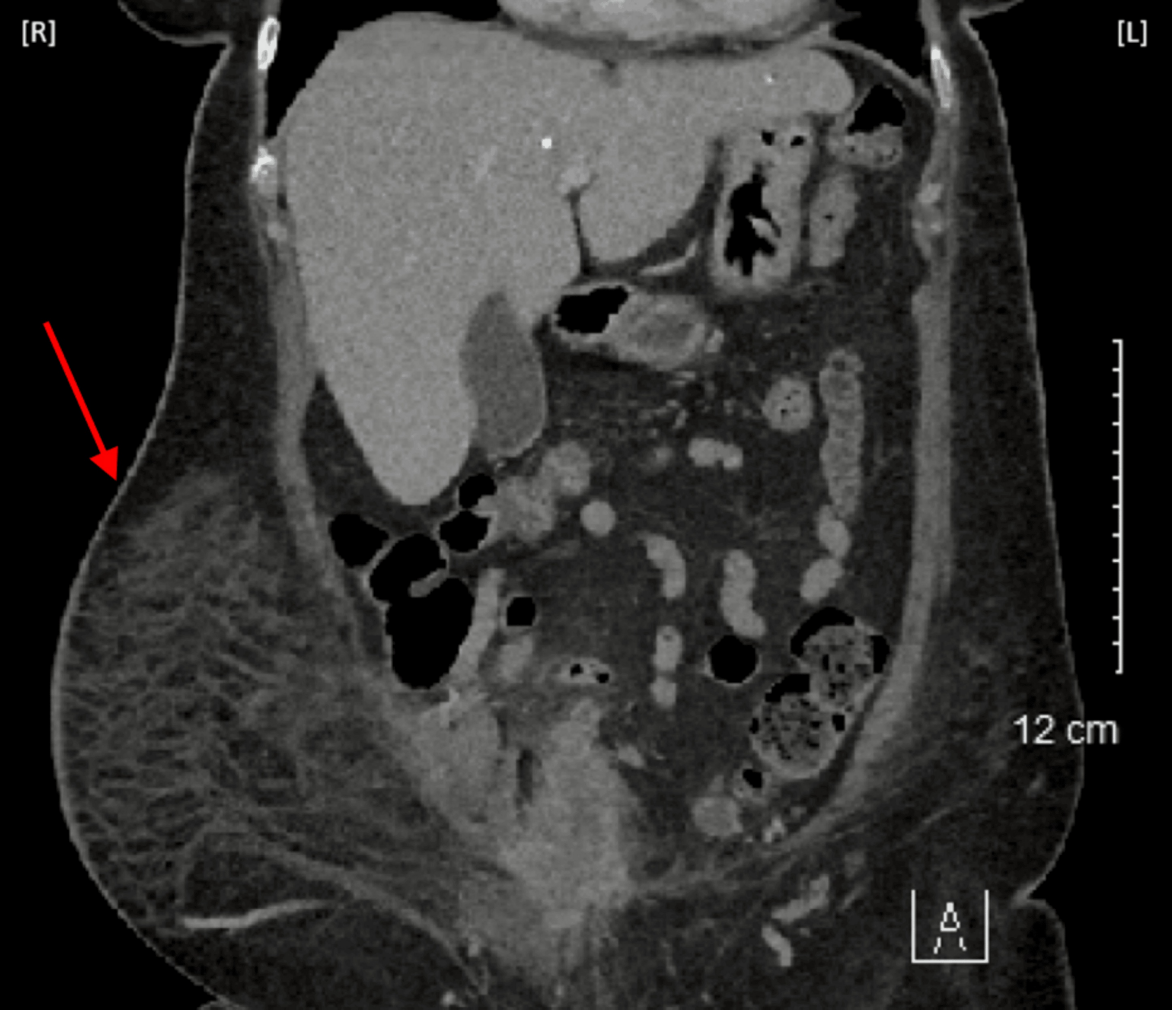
Coronal computed tomography scan of the abdomen and pelvis The red arrow identifies skin thickening with extensive subcutaneous fat stranding in the right lower quadrant of the abdomen. These findings correlate to an area of infection assisting in the diagnosis of necrotizing fasciitis. R: right; L: left
